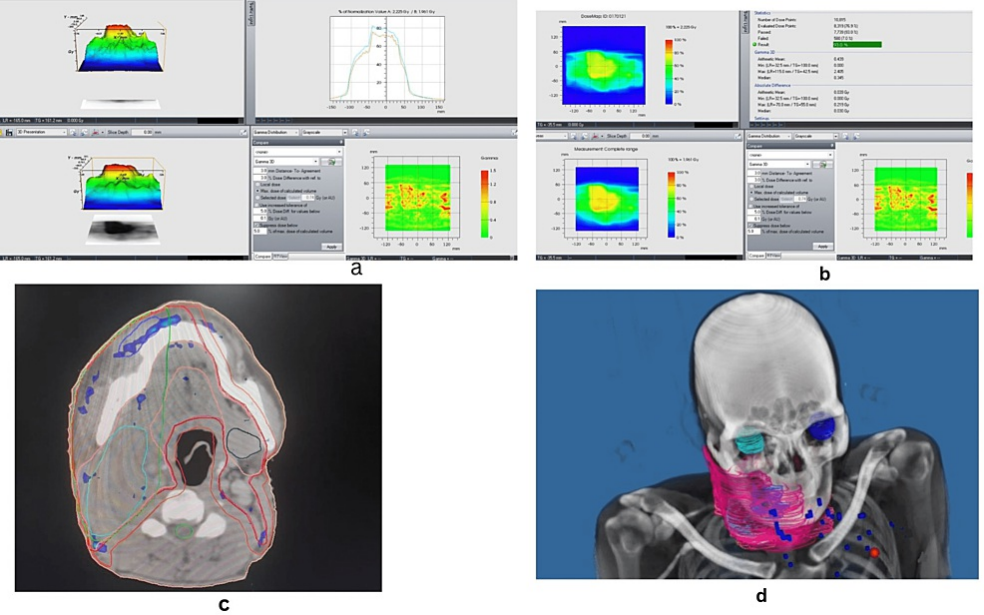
A patient diagnosed with buccal mucosa cancer. Image (a) shows a dosimetric comparison of TPS and verification plan and failing points in VeriSoft software. (b) 3D gamma index with 93% passing points with 3 mm/3% cut-off. Image (c) shows hotspots in the IMRT treatment plan (>107%). (d) CT overlay showing failing points in gamma analysis The areas of “lack of agreement” in gamma analysis should be interpreted in the context of patient anatomy for clinical decision-making, otherwise, it may potentially mask clinically relevant errors. TPS: treatment planning system; IMRT: intensity-modulated radiotherapy; 3D: three-dimensional.
